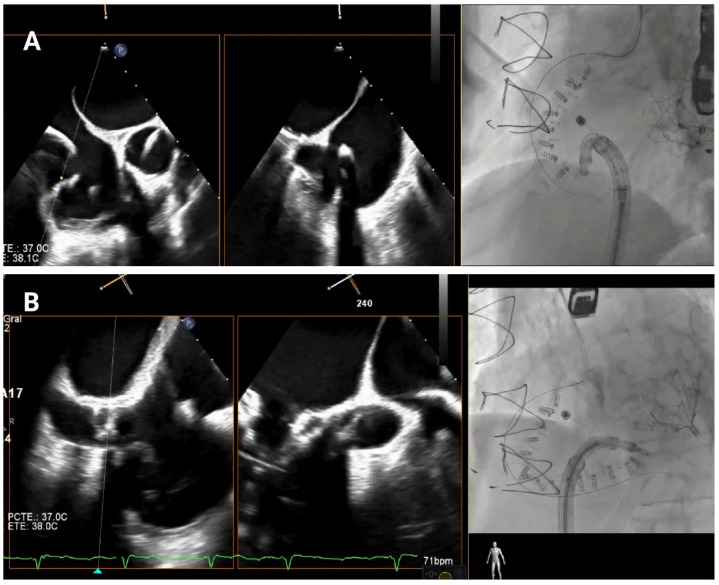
Posterior tricuspid annulus anchoring. (A) Posterior annulus anchoring requires navigating anatomical complexity due to echo beam alignment and distance from the probe. A 90° “hooking” angle between the implant catheter and the transvenous steerable sheath is often necessary to overcome the right coronary artery and access the posterior annular region. Transgastric transesophageal view (150°) and upper esophageal retroflexed views aid visualization, while RAO and LAO fluoroscopic projections confirm alignment and proper catheter trajectory. (B) Final anchoring at the posteroseptal commissure using deep and mid-esophageal bicaval views (0–135°) for visualization of the coronary sinus and septum. RAO projection and biplane imaging help verifying proper anchor placement and avoiding overlap or misalignment with nearby structures.
